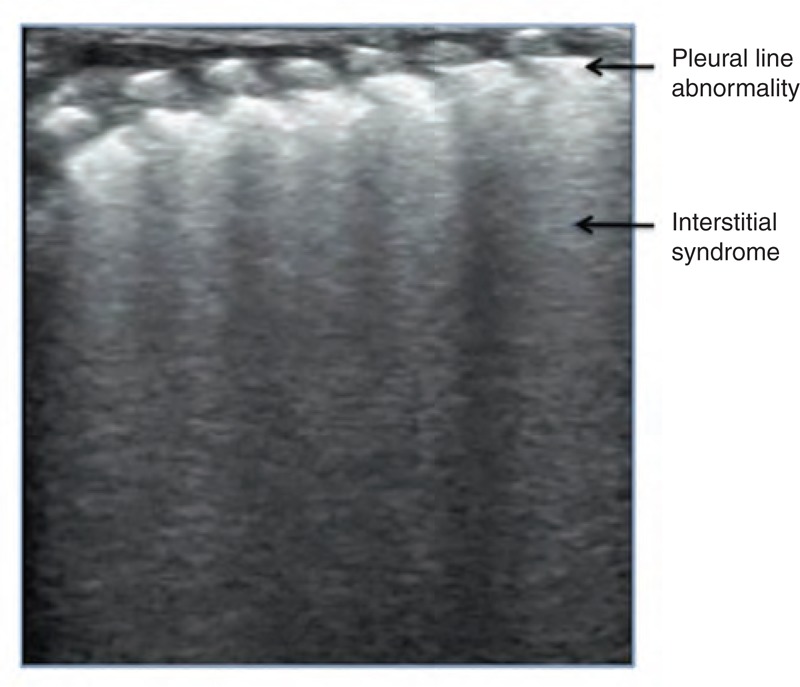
Lung edema. GA 27^+3^ weeks, birth weight 1000 g. This baby presented without spontaneous breathing after birth and was placed on a ventilator because of dyspnea following resuscitation. The patient was diagnosed with BPD clinically because oxygen therapy was required after 40 days of life. A lung ultrasound demonstrated a “white lung” appearance, as well as the coarse appearance of the pleural line in the right lung, suggesting the presence of severe pulmonary edema, which was the reason for this baby's LTOD. BPD = bronchopulmonary dysplasia, GA = gestational age, LTOD = long-term oxygen dependence.
